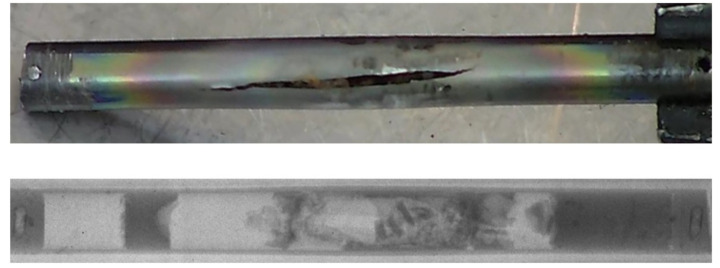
Photograph and radiography of damaged target rod (outer diameter approximately 10 mm) with remaining lead filling.
